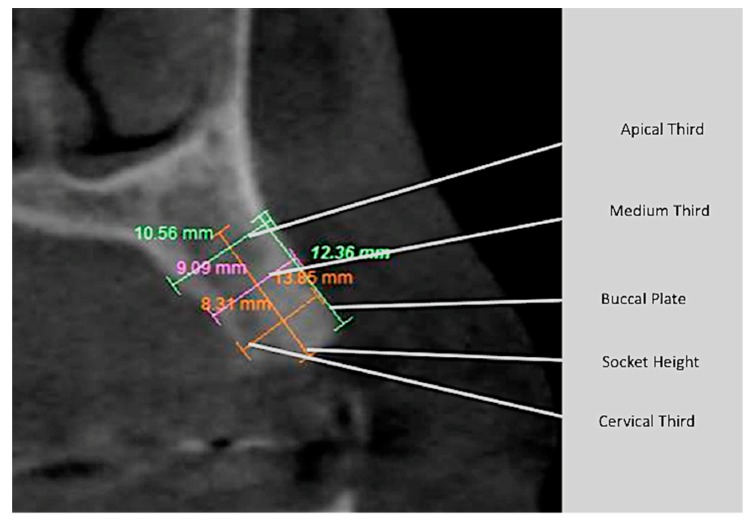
Scheme of the CT measurements, using DentalSlice software.
